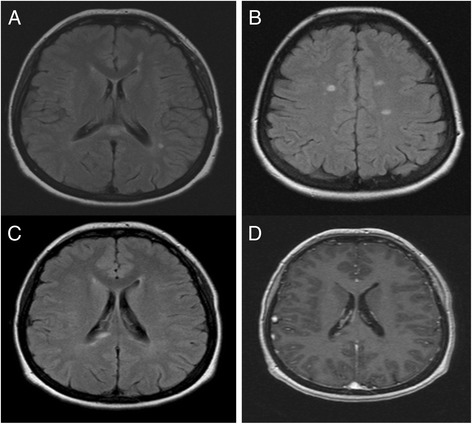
Brain MRI lesions of 52 year-old patient with CAPS before and after treatment. Axial FLAIR brain MRI images showing well limited periventricular (a), corpus callosum (a) and juxtacortical lesions (b). Brain MRI images with Axial FLAIR sequences (c) and T1 after gadolinium injection sequences (d), after one year of canakinumab treatment, of the same patient showing new FLAIR right paraventricular abnormalities with Gadolinium enhancement
